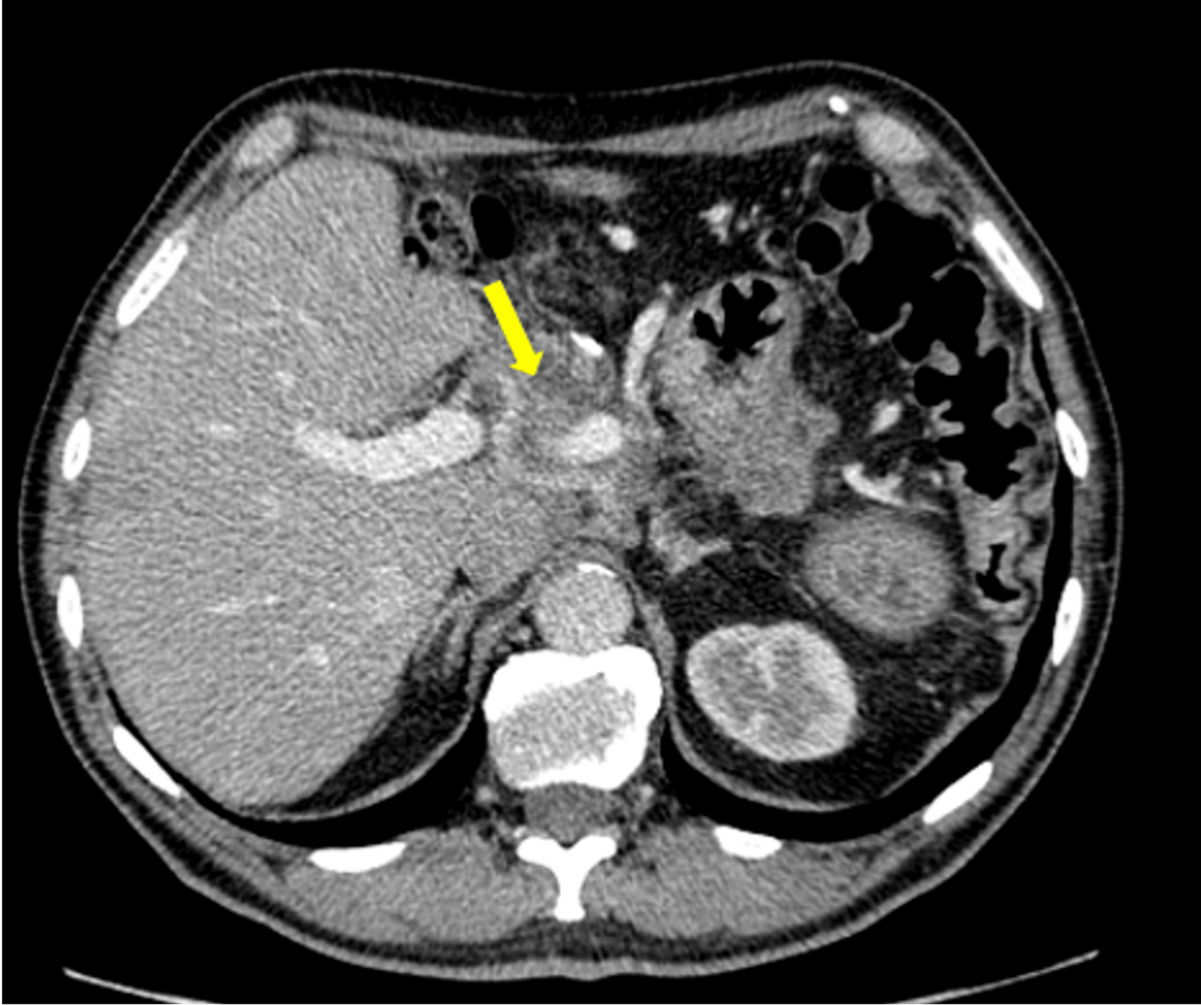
Axial CT performed in 2013 showing subtle soft-tissue density around the celiac axis, initially raising concern for recurrence (yellow arrow). Subsequent serial imaging confirmed stability of these postoperative changes with no radiologic, clinical, or biochemical evidence of disease recurrence.
